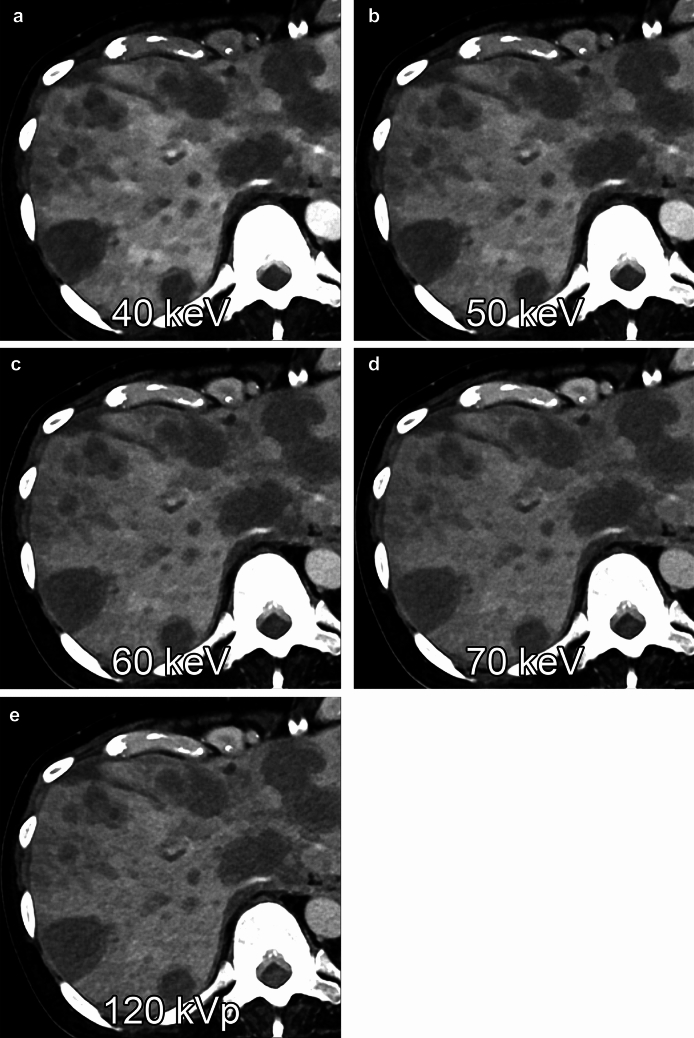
Multiple metastatic liver tumors from pancreatic cancer were shown on virtual monochromatic images at 40 (A), 50 (B), 60 (C), and 70 keV (D) and polychromatic image (*i.e.*, T3D) acquired with photon-counting CT at 120 kVp (E) during the portal venous dominant phase. The contrast-to-noise ratios between the liver metastasis and the liver parenchyma are 14.6, 11.9, 9.8, 8.5, and 7.4, respectively
